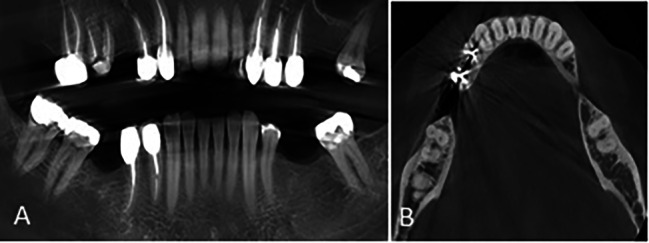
Mandibular second molar tooth with (A) type 1 morphology on the panoramic-like image and (B) double pattern on the axial CBCT section
